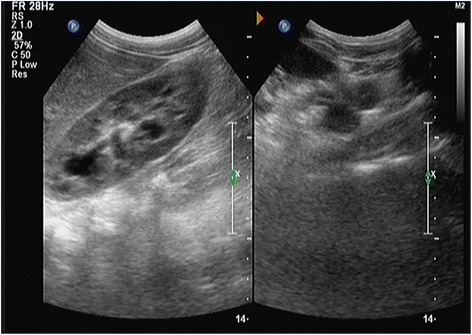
The follow-up abdominal ultrasound shows remarkably reduced right hydronephrosis
